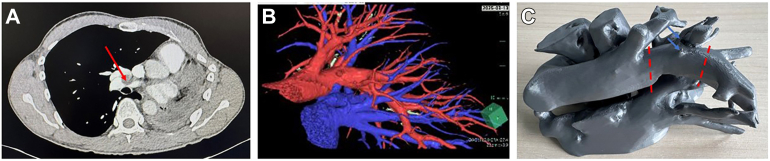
Preoperative planning of the surgery. (A) Preoperative computed tomography scan; the arrow depicts the sarcoma in the right pulmonary artery. (B) Three-dimensional reconstruction of the patient’s pulmonary artery. (C) Three-dimensional printed model derived from the reconstruction; the dashed lines depict the planned resection margins, and the arrows indicate the 2 A2 branches that had to be sacrificed.
